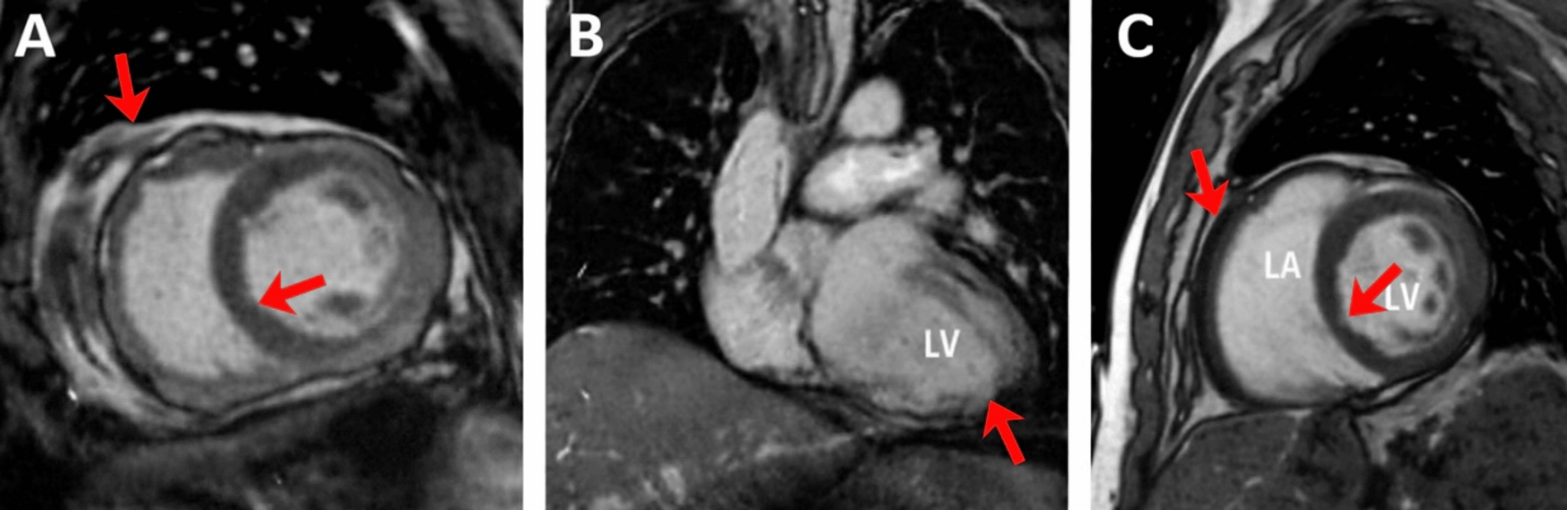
Cardiac Magnetic Resonance Imaging A: Short-axis view: a cross-sectional slice of the left ventricle (LV) and right ventricle (RV) at the mid-ventricular level (as indicated by arrows). The myocardial walls show uniform thickness without evidence of gross structural abnormalities. B: Coronal view: a longitudinal orientation showing the LV and the aortic outflow tract. The image displays normal cardiac positioning and the relationship between the major chambers and the ascending aorta (as indicated by arrows). C: Labeled short-axis view: a repeat of the short-axis perspective with the LV and the RV (labeled as 'LA' on the right side) cavities identified (as indicated by arrows). The myocardium appears dark (isointense), indicating a lack of focal hyperintensities or late gadolinium enhancement.
